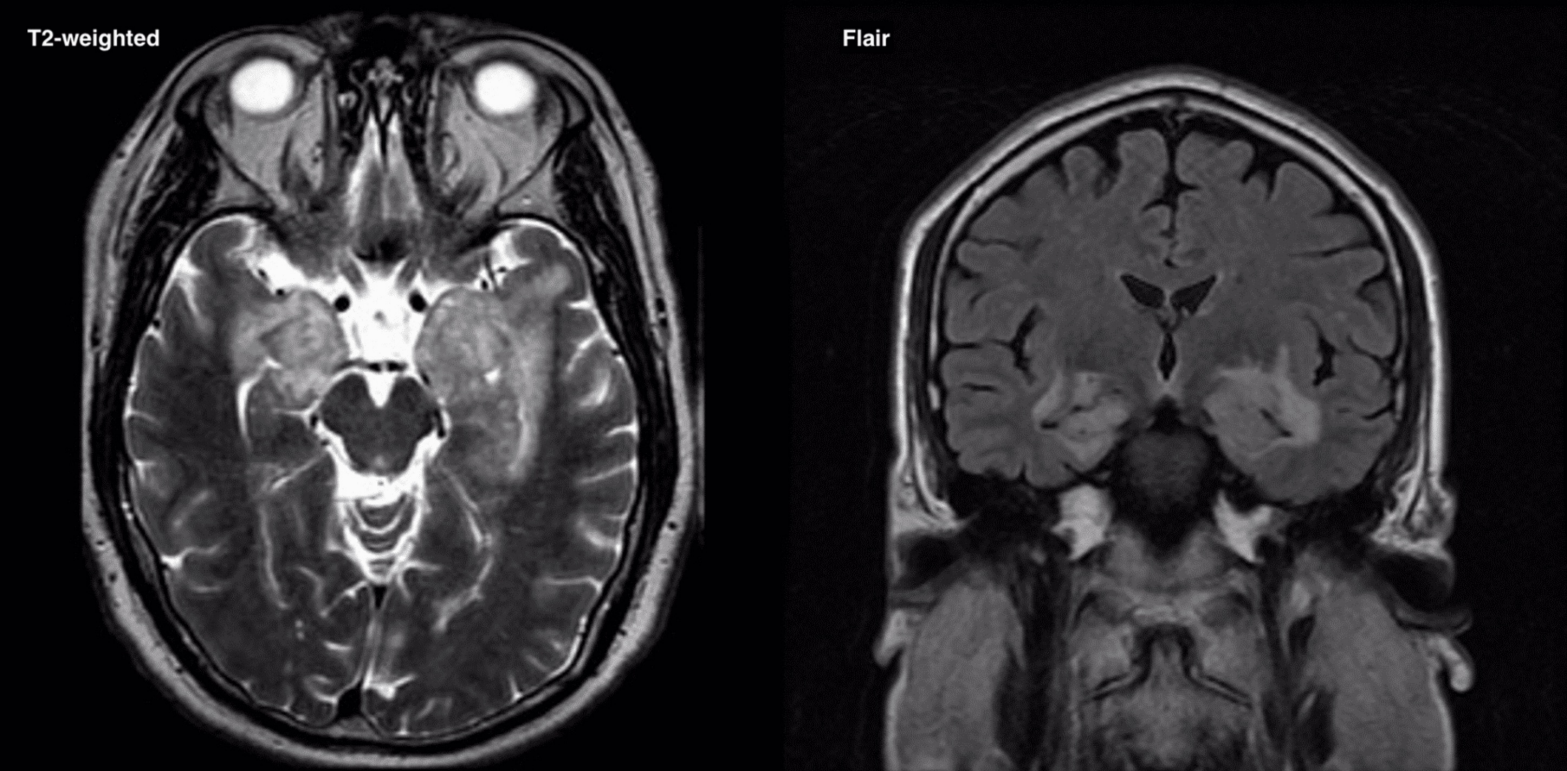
T2-weighted (left) and FLAIR MRI (right) Demonstrating relatively symmetric hyperintensities affecting both medial temporal lobes, involving the hippocampi, amygdala, hypothalamic, and optic chiasma FLAIR: Fluid-attenuated inversion recovery; MRI: Magnetic resonance imaging
